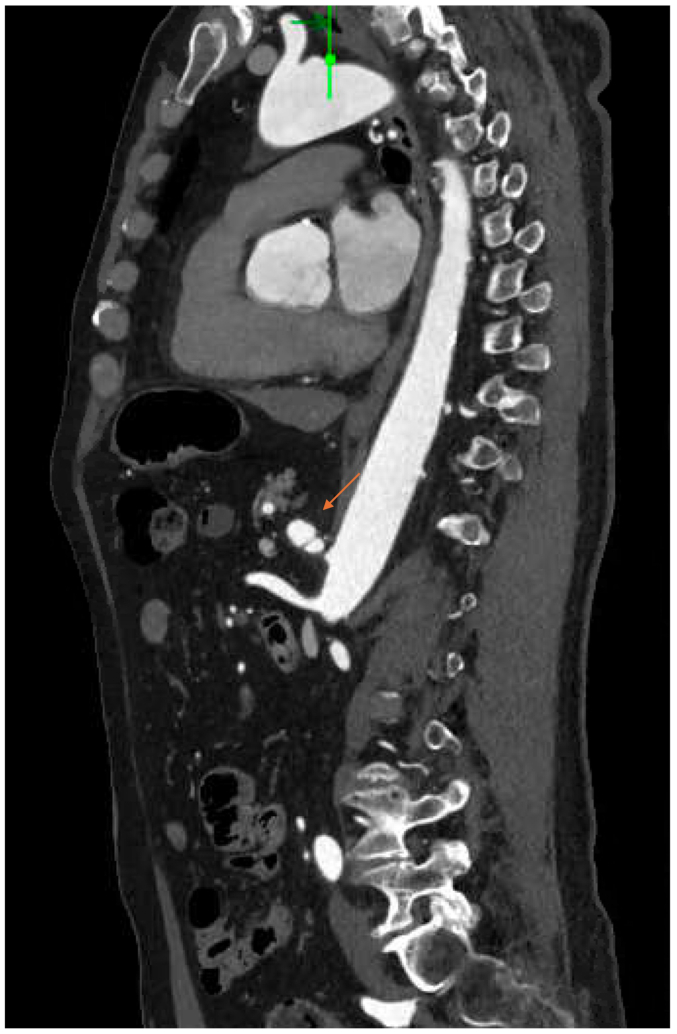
Computed tomography angiogram (CTA) sagittal view showing celiac artery dissection, *orange arrow*.
